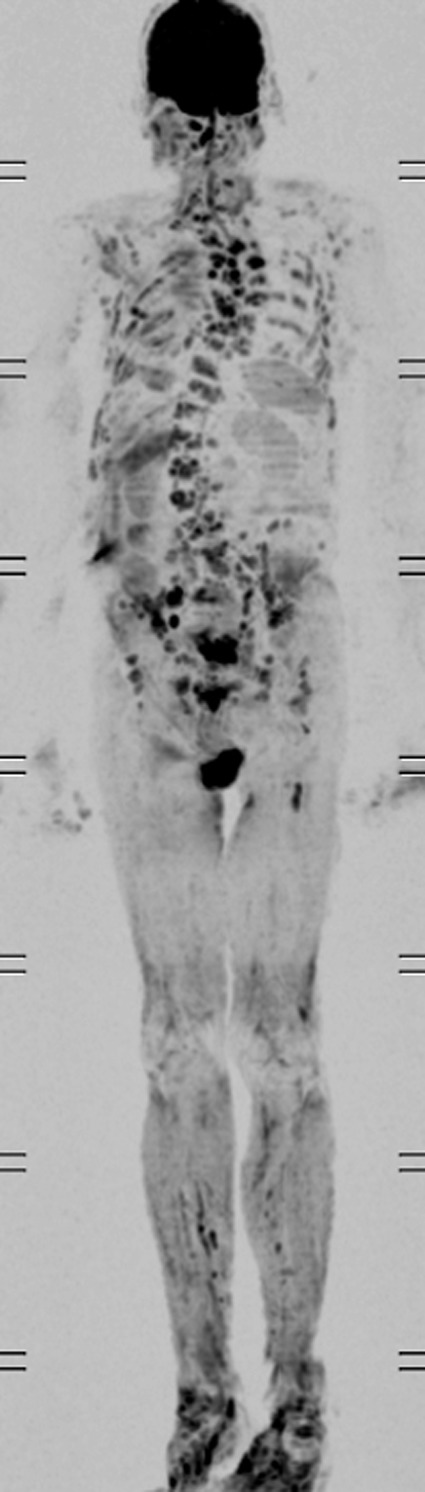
MRI DWIBS shows multiple lesions throughout the thoracic and lumbar spine. Differentiation between multiple myeloma and metastatic prostate cancer is difficult.
